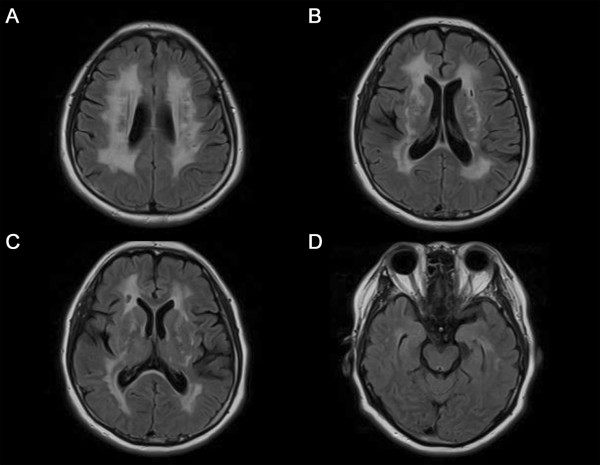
Cerebral MRI with fluid-attenuated inversion-recovery (FLAIR) showing high-signal intensity lesions in the periventricular white matter, both basal ganglia, thalamus, and external capsule (A-C), but not prominent in the anterior temporal poles (D).
